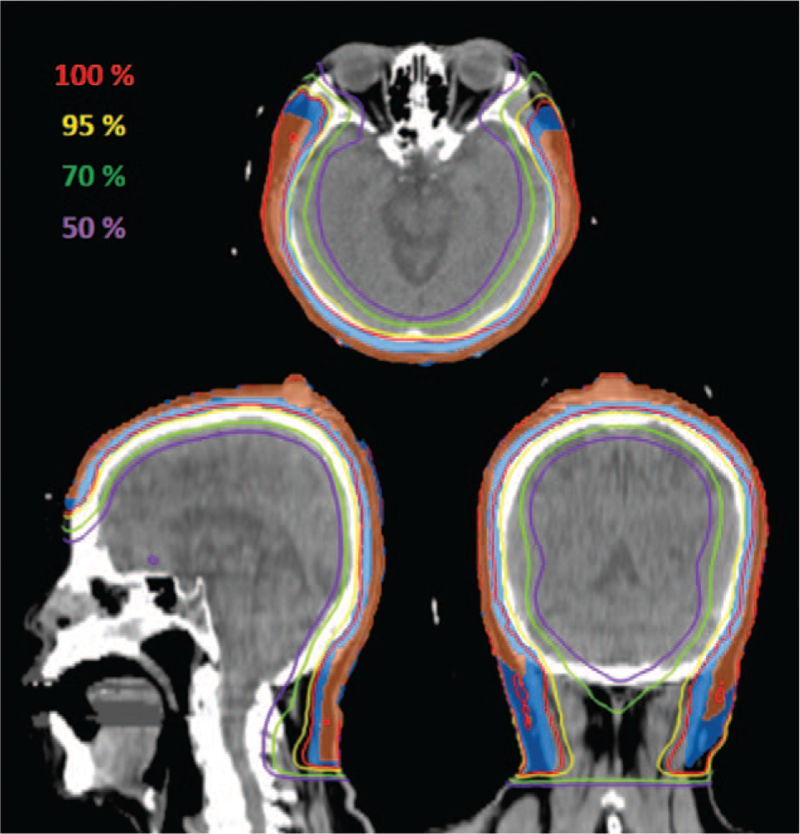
Dose distributions of helical tomotherapy. Axial, coronal, and sagittal views of computed tomography with superimposed dose distribution. The clinical target volume and planning target volume are rendered in orange and blue, respectively.
